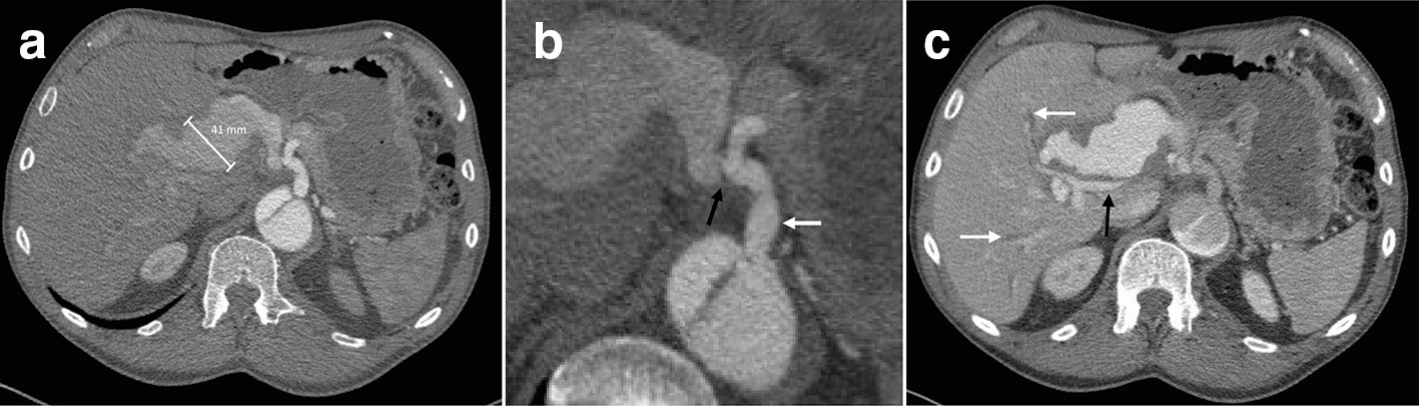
Repeat CT 1 week later, using the same imaging parameters as the previous scan with the addition of a portal venous phase performed 70 s following commencement of contrast injection. (a) The arterial phase study shows interval enlargement of the aneurysm to 41 mm; (b) zoomed axial image demonstrating the course of the coeliac artery (white arrow- please note this arrow has moved since the figure has been reformatted), with an intimal flap across the common hepatic artery origin (black arrow- please note this arrow has moved since the figure has been reformatted). (c) The portal venous phase study shows compression of the portal vein (black arrow) and intrahepatic biliary dilatation (white arrows).
